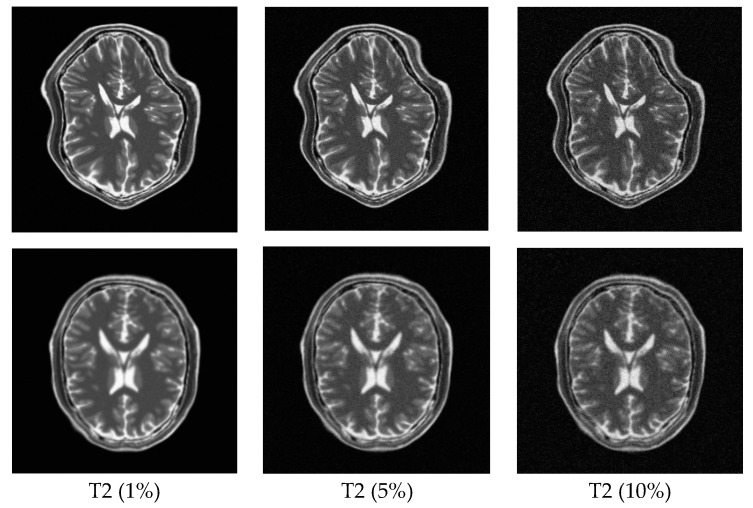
Registration results for T1–T2 images with different levels of Rician noise. The first row shows the T2-weighted floating images with 1%, 5%, and 10% Rician noise, respectively. The second row corresponds to the registered results for the PSR method.
